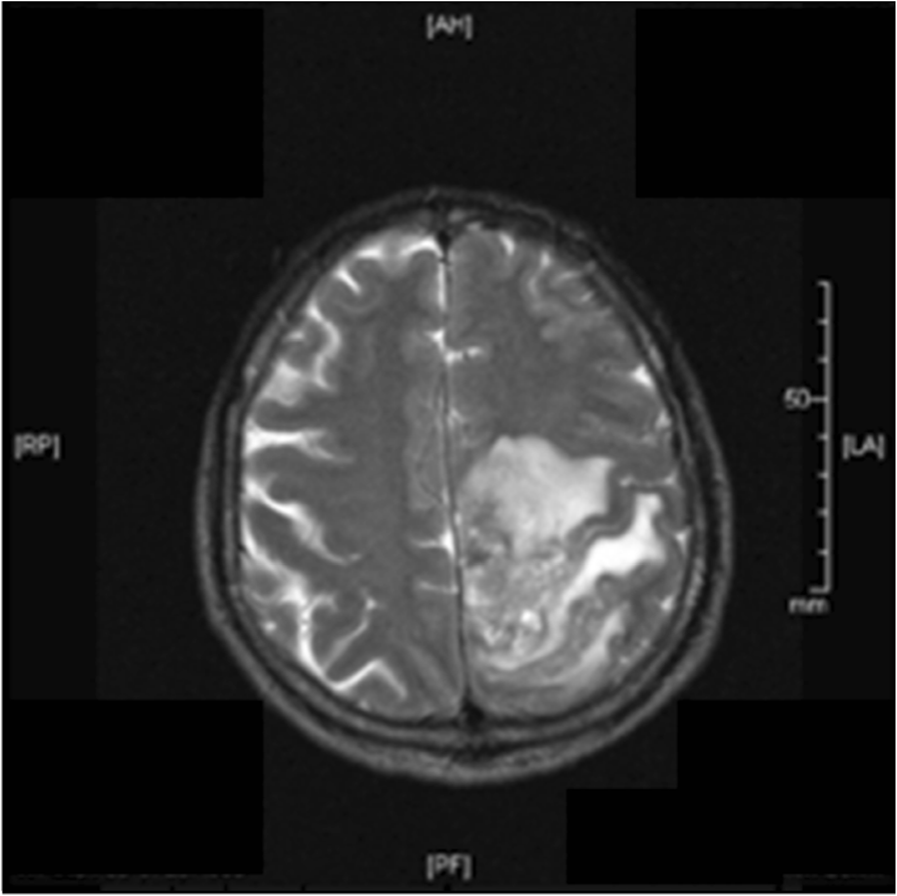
Magnetic resonance imaging on day 6 with pronounced perifocal edema in the left parieto-occipital region
